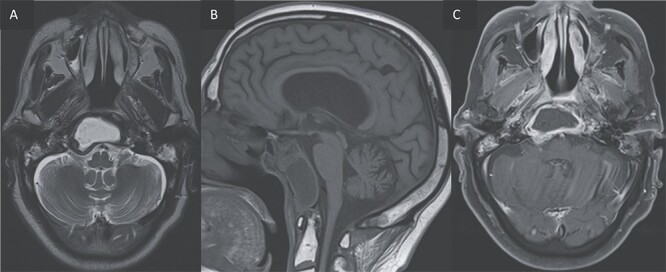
(A, B) Axial T2 and sagittal T1 images showing an expansile cystic lesion in the center of the clivus hyperintense in T2, hypointense in T1, with a rim hypointense in T2-weighted image. (C) Post-gadolinium T1-weighted shows a peripheral rim enhancement of the clival lesion.
